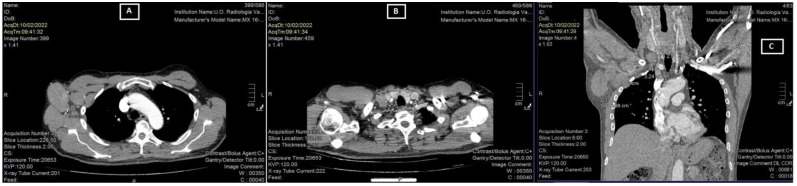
CT scan revealed two lymphadenopathies: right axillary 6.99 cm (A) and supraclavicular 2.36 cm (B), and multiple adenopathies in the coronal section (C).
